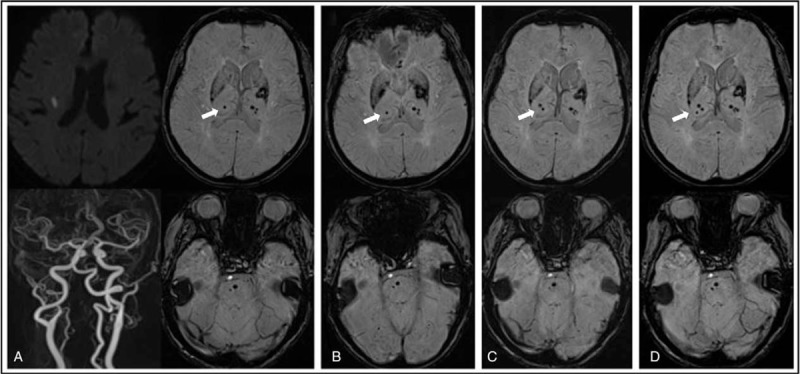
Brain images of the patient. (A) Brain MRI with acute ischemic stroke. Brain DWIs show right lenticulostriatal artery territorial infarction. Multiple microbleeds are seen in the bilateral deep gray matter (arrow) and pons on GREs. (B) The newly taken DWI and GRE brain MRI do not appear different from previous images (arrow). (C) Eight months after the previous ischemic stroke, a new microbleed was observed (arrow) in addition to previous microbleeds in the right thalamus on GRE, with the patient presenting with left hemiparesis and paresthesia. (D) Two months after the previous microbleed, a new microbleed was observed (arrow) in addition to previous microbleeds in the right thalamus on GRE, with the patient again presenting with left paresthesia. DWI = diffusion-weighted image, GRE = gradient echo image, MRI = magnetic resonance imaging.
